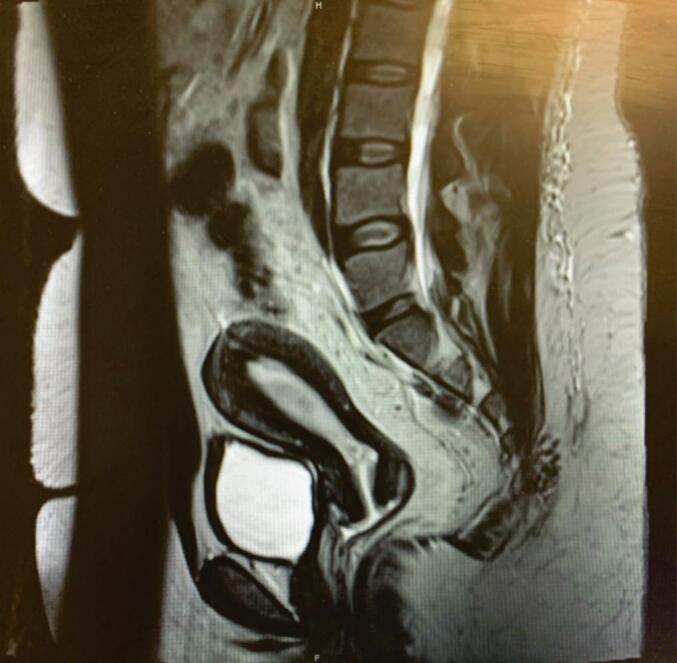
Vaginal Strictures visualized on MRI pelvis with and without contrast.
